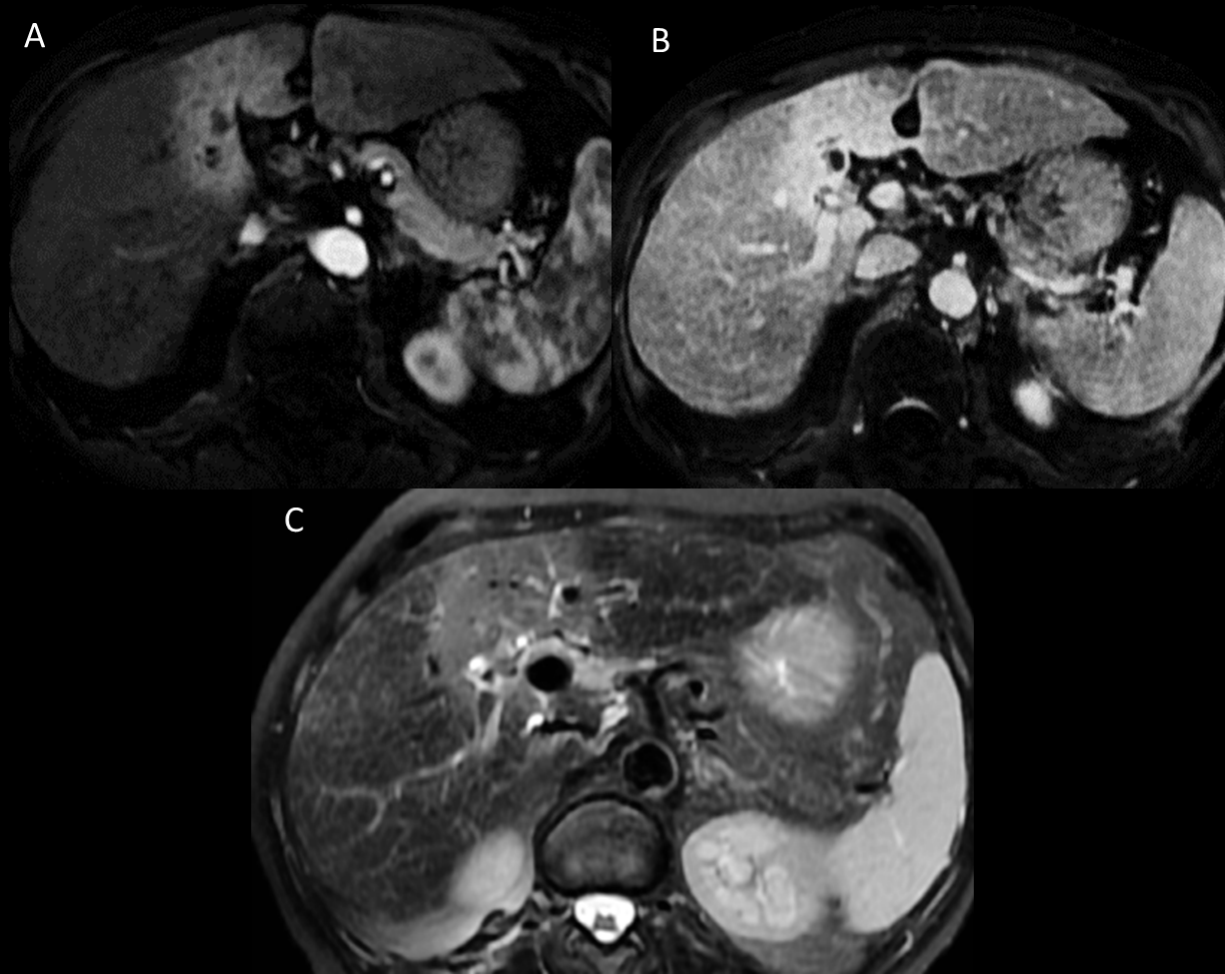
A 48-year-old man with HCC. MRI at three months. 4A Enhanced post-treatment changes on arterial phase. 4B. Enhanced post-treatment changes on venal phase. 4C. T2-weighted hyperintense post-treatment changes.
